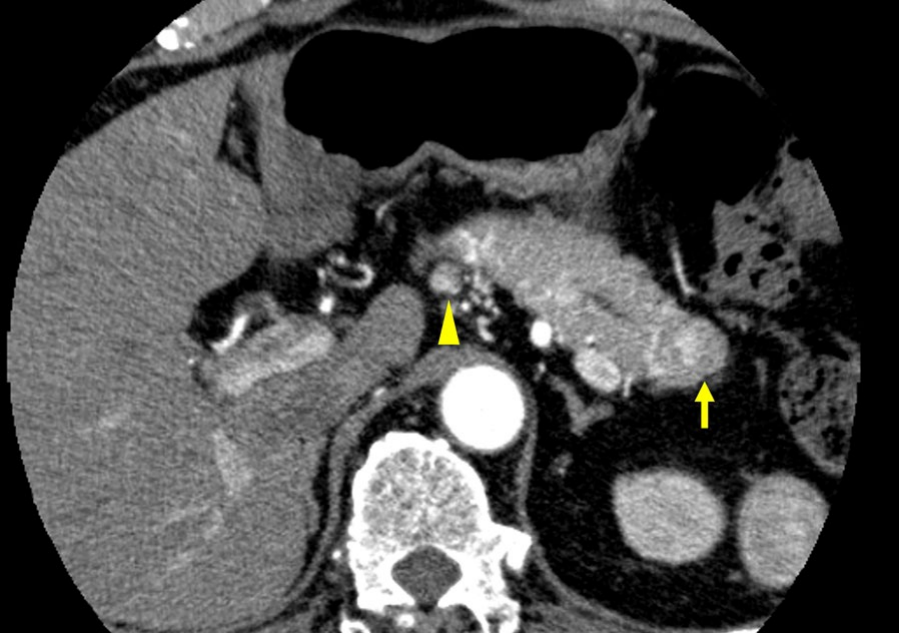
Contrast-enhanced computed tomography images before the operation. Hypervascular lesions were identified in the pancreatic parenchyma (yellow arrow), and a regional lymph node was swollen (yellow arrowhead)
